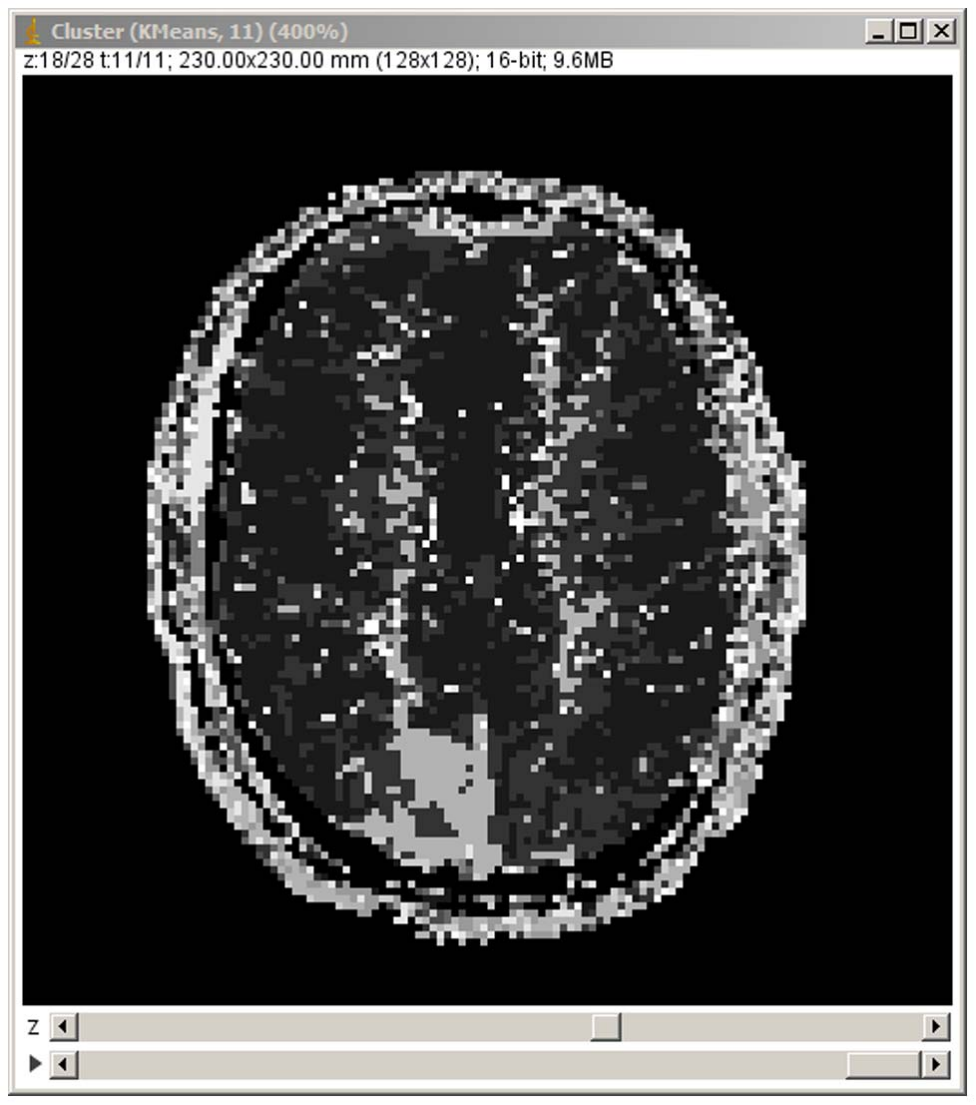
Example of the tool applied to a dynamic magnetic resonance study. Clustering results for a dynamic MRI study using gadolinium as a contrast agent in a human subject. A lesion can be seen clearly at the bottom of the image. In this case, the full image screen is shown to highlight the ImageJ frame and the dynamic image controls that allow the user to switch between the different classes or, as in this case, a last frame containing all the classes, each with a different gray level.
